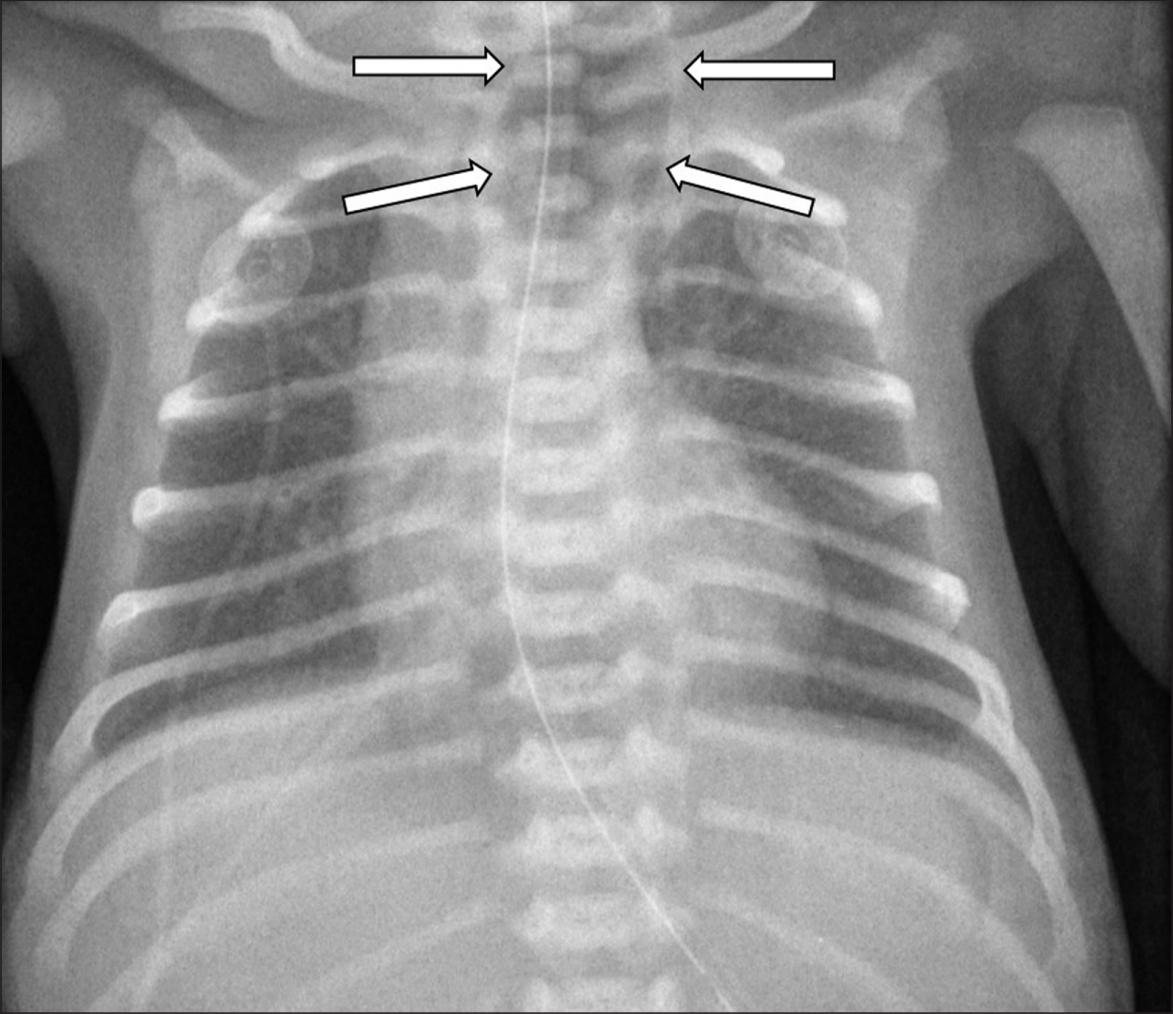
Thoracic X-ray shows a progressive widening air column (white arrows)
